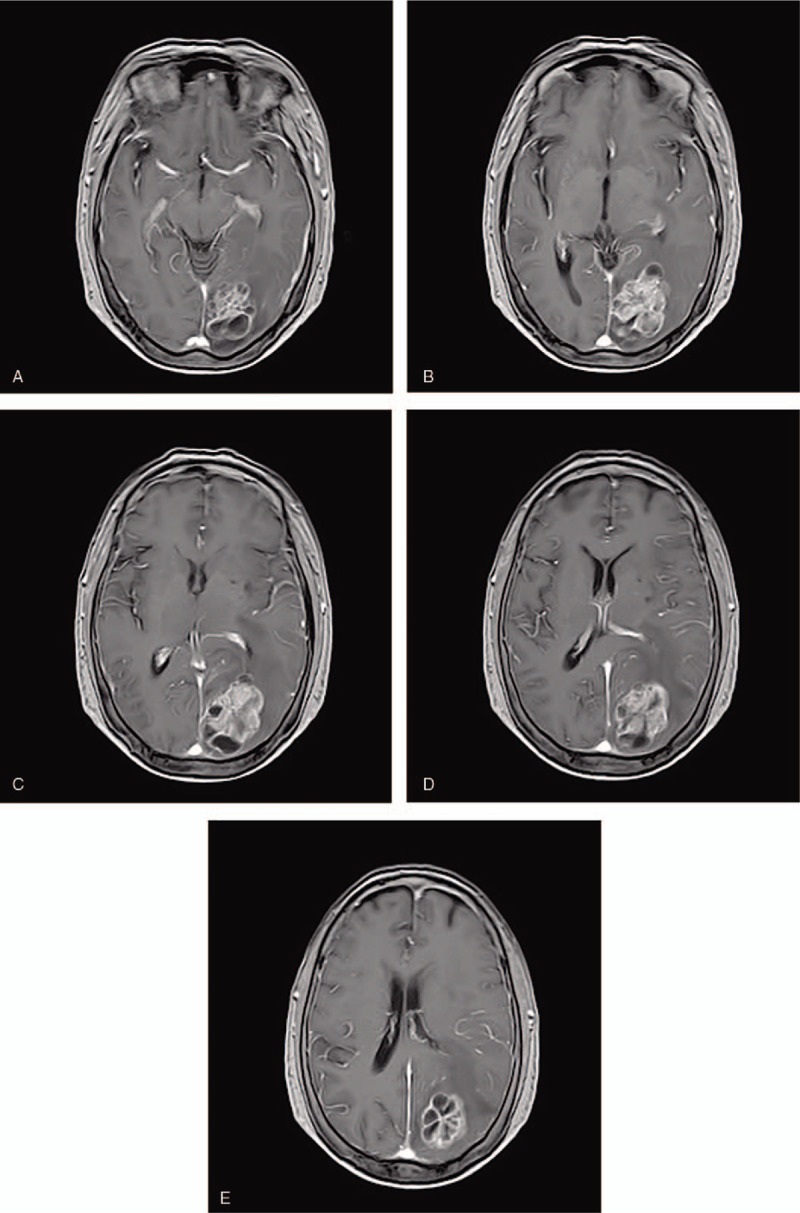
(A–E) Magnetic resonance imaging of the patient with diagnosis of left occipital metastases.
